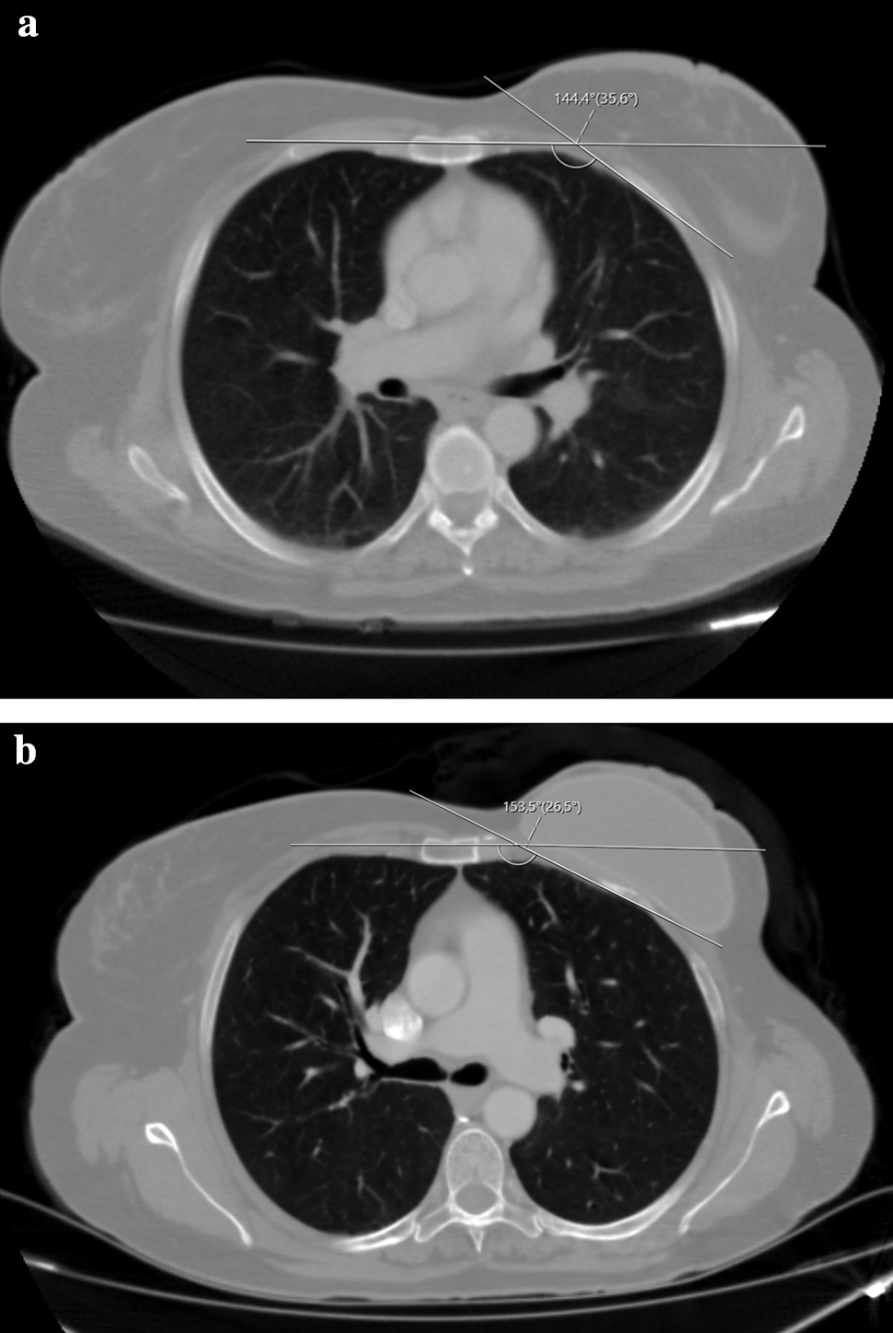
Picture a : PET-CT imaging reveals the anatomical condition of a patient during the preoperative evaluation preceding the initial stage of expander surgery. Picture b: taken subsequent to the second-stage expander surgery with a permanent implant, a notable observation is a 9.1° flattening of the fourth rib compared to its appearance between the two stages of surgical intervention
